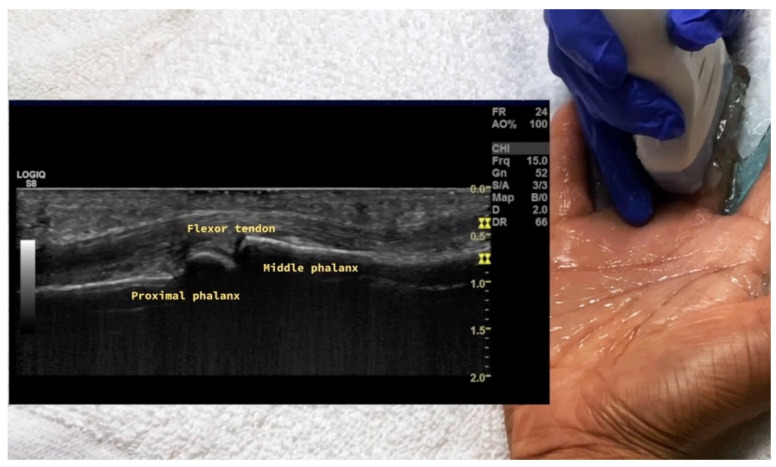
Probe placement and ultrasound of volar longitudinal view of MCP joint. MCP = metacarpal phalangeal.
